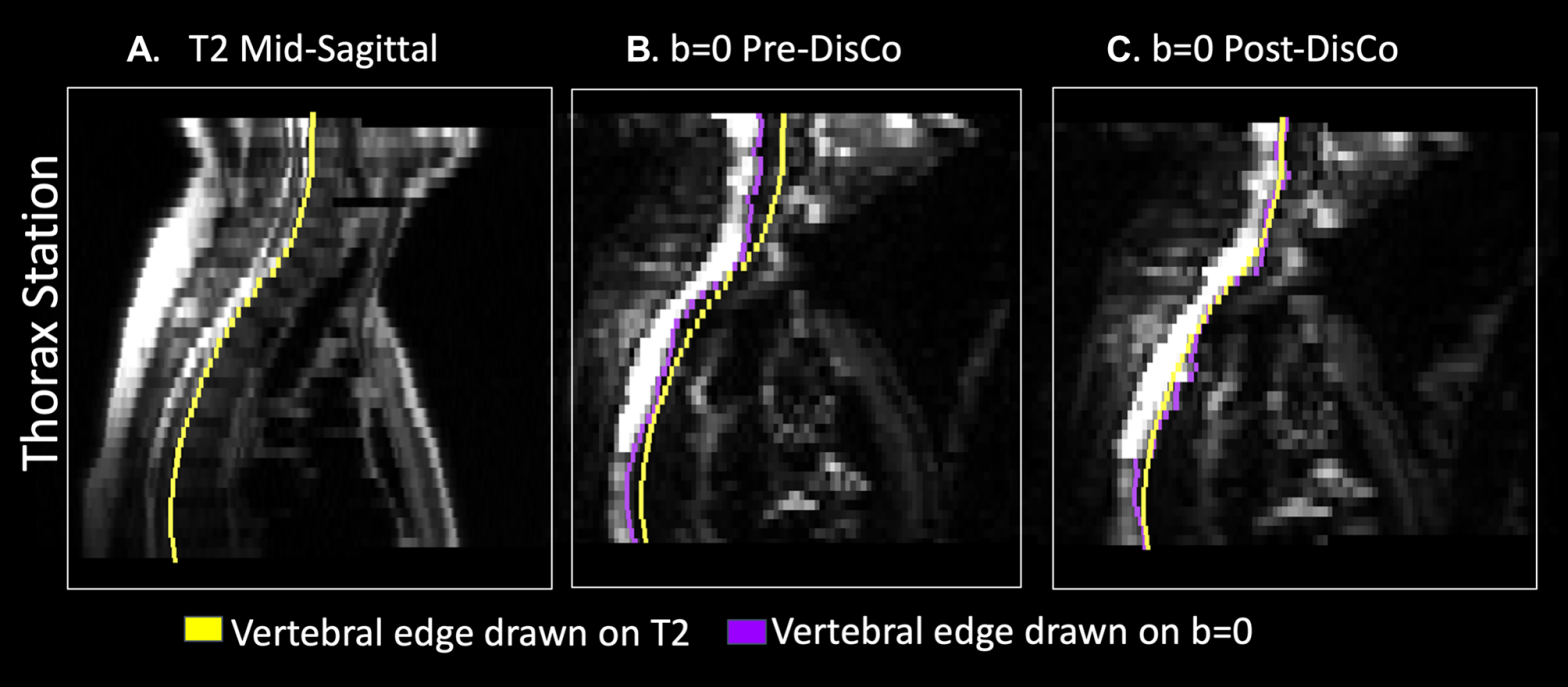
Example of tracing of posterior border of vertebrae for error change analysis. (A) Mid-sagittal slice of T2-weighted MRI from the Thorax Station for a single subject. The yellow represents the annotation of the posterior edge of the vertebral column on the T2. (B) Mid-sagittal slice of the DWI b = 0 s/mm^2^ prior to DisCo. The purple in this panel represents the annotation of the posterior edge of the vertebral column on the pre-DisCo DWI b = 0 s/mm^2^. (C) Mid-sagittal slice of DWI b = 0 s/mm^2^ after DisCo. The purple in this panel represents the annotation of the posterior edge of the vertebral column on the post-DisCo DWI b = 0 s/mm^2^. The average distance between the yellow and purple tracings (i.e., the error) decreases after application of DisCo. *DisCo* distortion correction.
